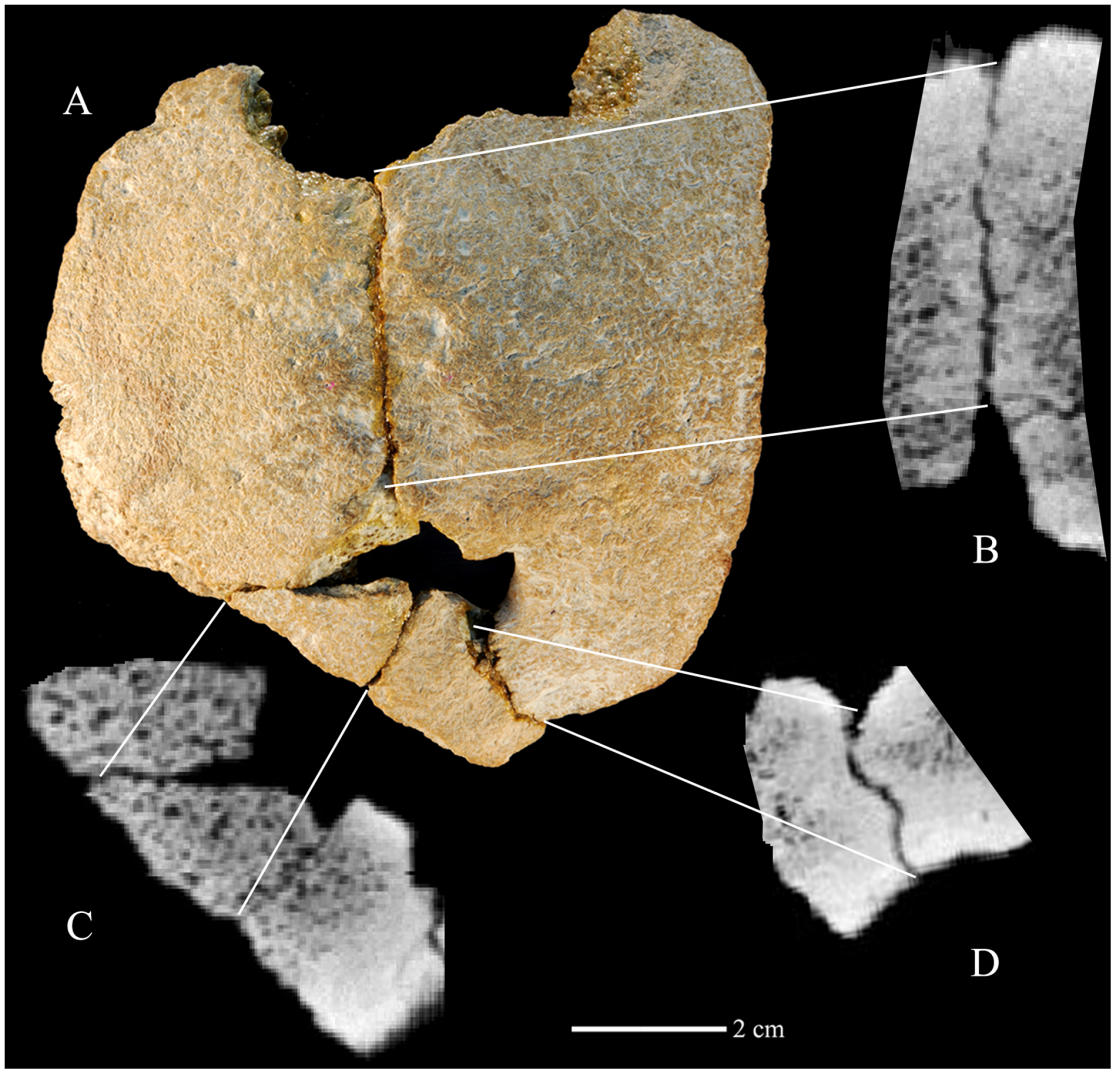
The Xujiayao 11 parietal bones. Exterior view (A). CT horizontal section images showing the linear sagittal suture (B), the posterior left postmortem breaks (C), and the posterior right oblique sagittal suture (D). Anterior is above.
